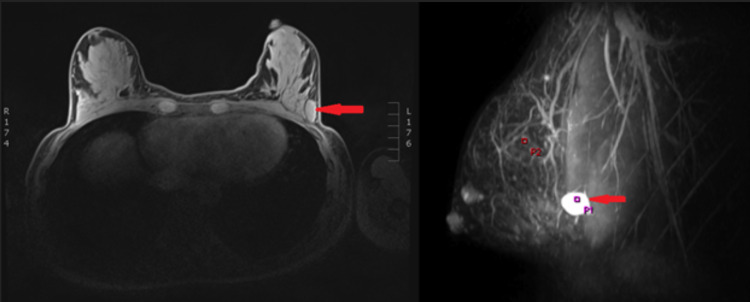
Bilateral MRI breast demonstrating the breast DFSP MRI demonstrated a predominantly benign enhancement; however, the central portion showed an area of increased vascularity with a very rapid enhancement kinetic curve. The lesion was seen in the subcutaneous tissue, superficial to the pectoral fascia, and the mass exhibited a high signal intensity on the T2-weighted images (arrows) MRI: magnetic resonance imaging; DFSP: dermatofibrosarcoma protuberans
